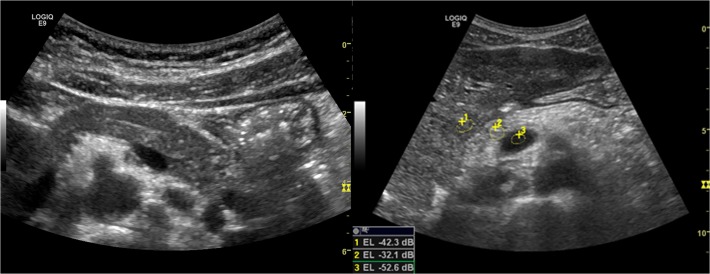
Pancreas ultrasonography. The figure is displaying a normal pancreas in pancreas sufficient patient (left) and a typical hyper echoic pancreas in an insufficient patient (Right). The Visual score graded echogenicity 1 for the left pancreas and 4 for the right pancreas. The image to the right display region of interest (ROI) for Echo intensity measures (1: Liver, 2: pancreas, 3: Vessel (superior mesenteric vein)).
